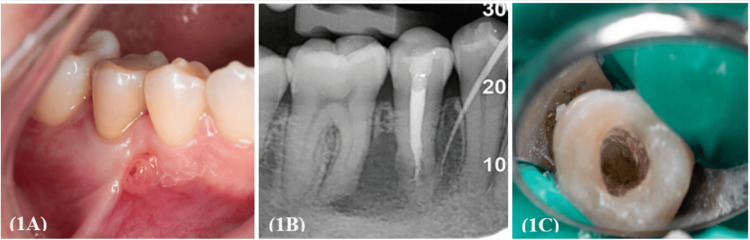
Pre-operative clinical and radiographic findings with access cavity preparation (1A) Presence of intraoral swelling and sinus tract in relation to tooth 45. (1B) Pre-operative intraoral periapical radiograph revealing a well-defined periapical radiolucency, with sinus tract tracing localized to tooth 45. (1C) Access cavity preparation performed under rubber dam isolation.
